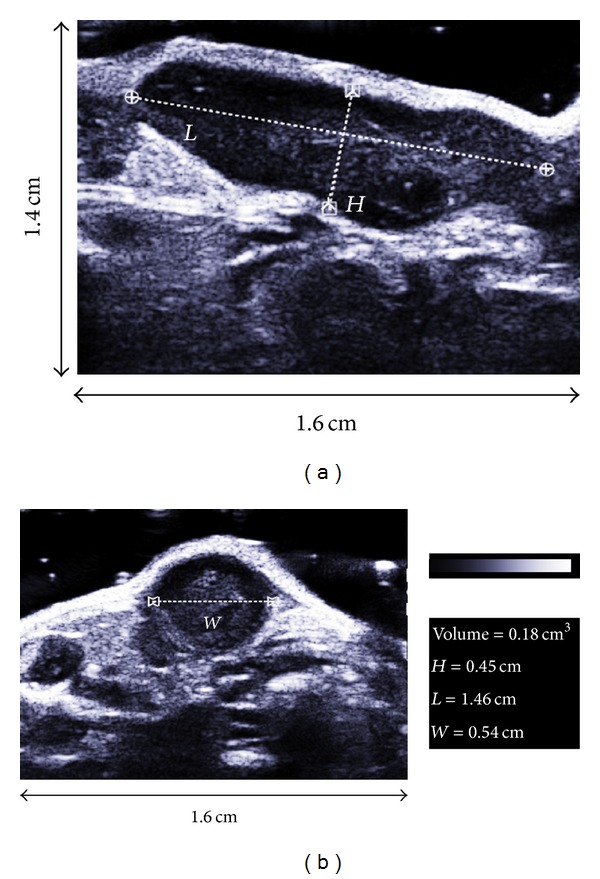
Method used for the calculation of tumour volume by ultrasound imaging. Images show an example from an ectopic tumour on day 15 using a saggital (a) and an axial (b) view.
